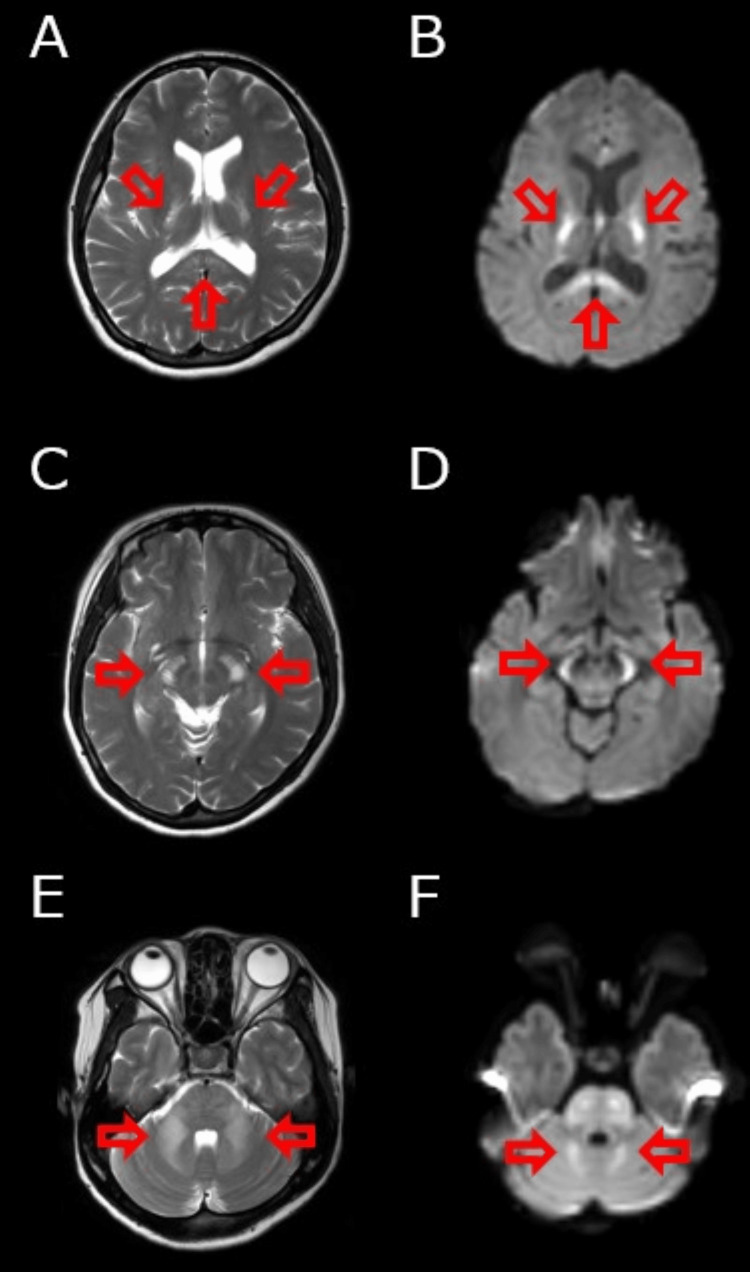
Brain magnetic resonance imaging of the patient at the time of presentation Hyperintensities on axial T2-weighted imaging (A, C, E) and diffusion-weighted imaging (B, D, F). Abnormal signals in the bilateral posterior limbs of the internal capsule and splenium of the corpus callosum (A, B), cerebral peduncle (C, D), and superior cerebellar peduncles (E, F).
